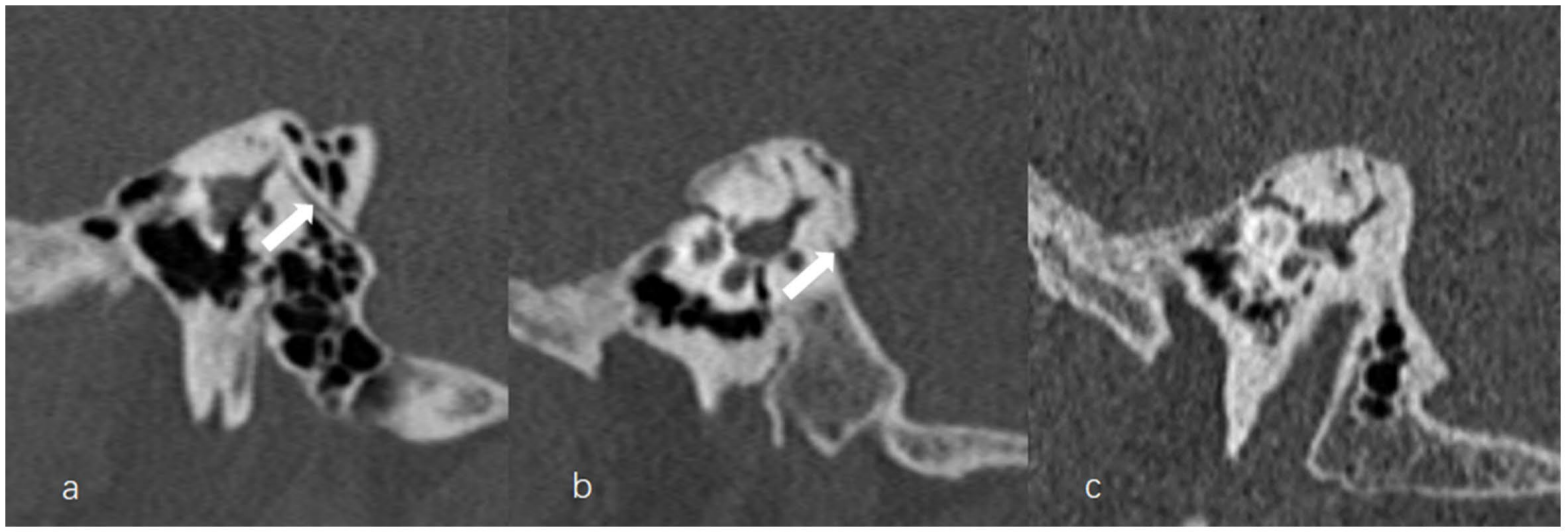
The grading of vestibular aqueduct (VA) visibility on the 45° oblique (Pöschl) planes on temporal bone computed tomography. (a) Grade 0 with a continuous VA (arrow). (b) Grade I with a discontinuous VA (arrow). (c) Grade II with a complete ossification of VA.
